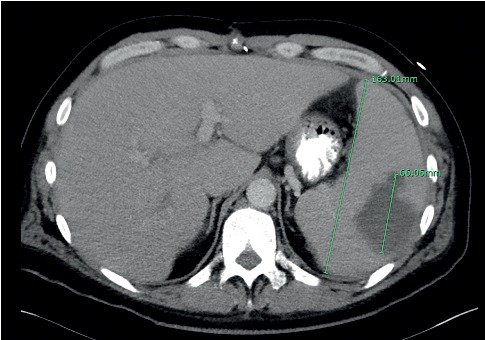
CT scan of the abdomen and pelvis with contrast showing splenic infarct with large splenic abscess collection in size of 66.05 mm and the enlarged spleen in size of 163.01 mm.
